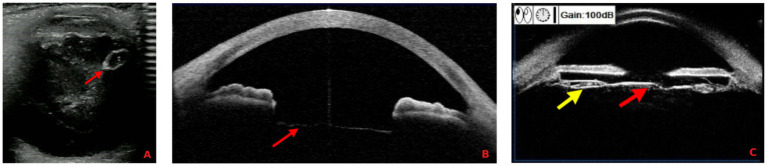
(A) Preoperative B-scan ultrasonography of the right eye. The lens nucleus is indicated by the red arrow. (B) Preoperative AS-OCT of the right eye. The intact anterior capsule is indicated by the red arrow. (C) Preoperative UBM of the right eye. The anterior capsule is indicated by the red arrow, and the ruptured posterior capsule is indicated by the yellow arrow.
